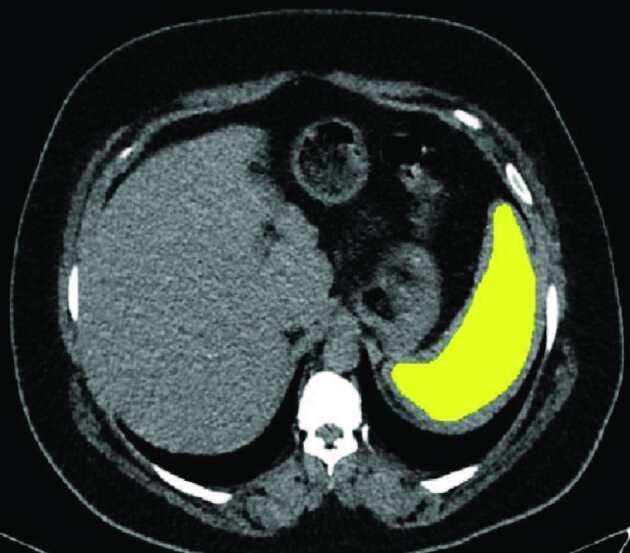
Axial nonenhanced CT showing the region of interest [ROI] was drawn manually to cover the entire spleen parenchyma. Care was taken not to extend the ROI limits a few millimeters from the periphery of the spleen parenchyma to avoid possible errors.
